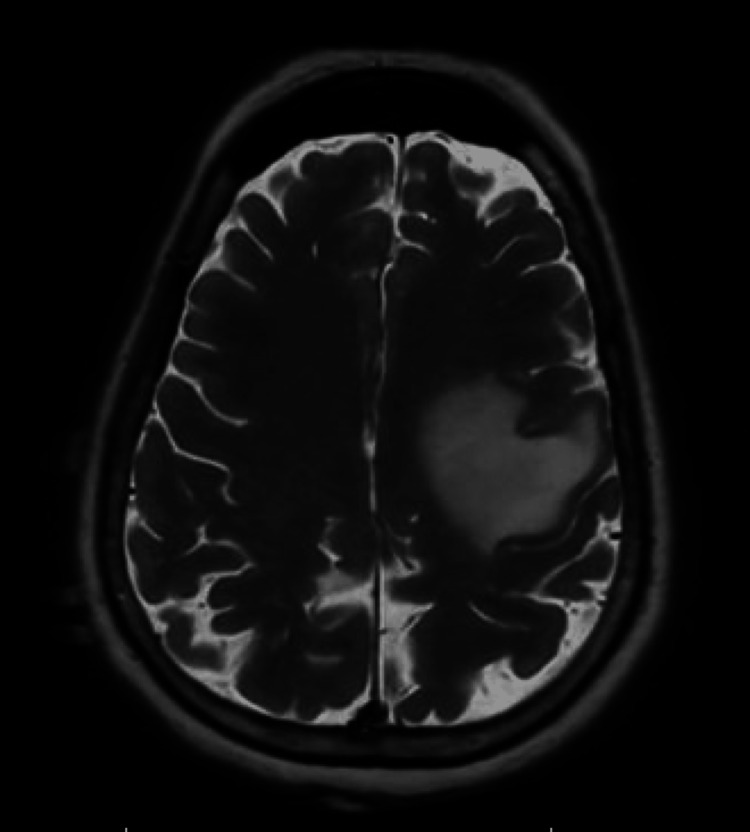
MRI brain: The T2-weighted image shows moderate to marked secondary vasogenic edema, and there is effacement of overlying cortical sulci
